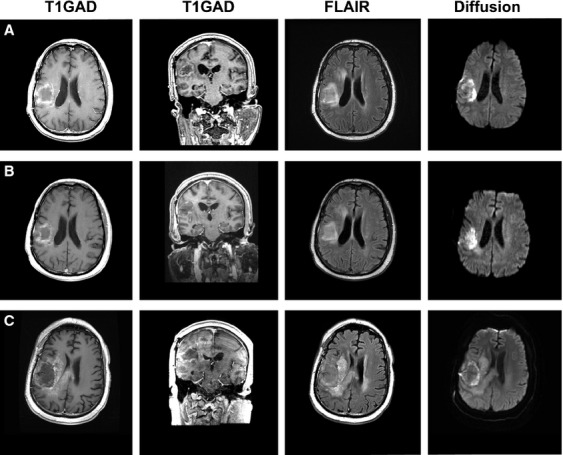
Stable disease from NovoTTF-100A, bevacizumab and TCCC. (A) At baseline, the necrotic recurrent glioblastoma in patient 3 was located in the right insula before treatment. (B) At 0.8 months after initiation of treatment, there was a slight reduction in gadolinium enhancement but no shrinkage of the tumor cyst. (C) At the time of tumor recurrence 6.4 months after initiation of treatment, his head MRI showed marked tumor invasion into the adjacent hemisphere and the corpus callosum.
